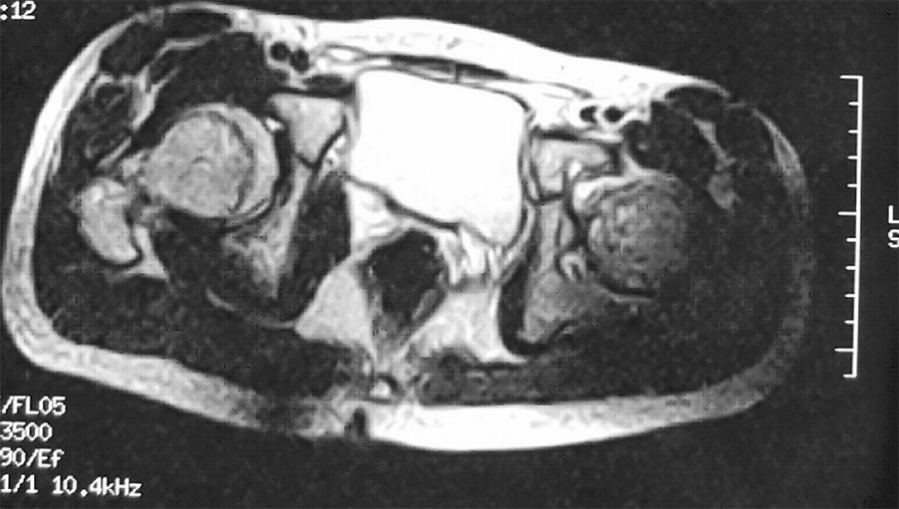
The horizontal MRI of bilateral hip joints, the femoral head and acetabular articular surfaces were rough
